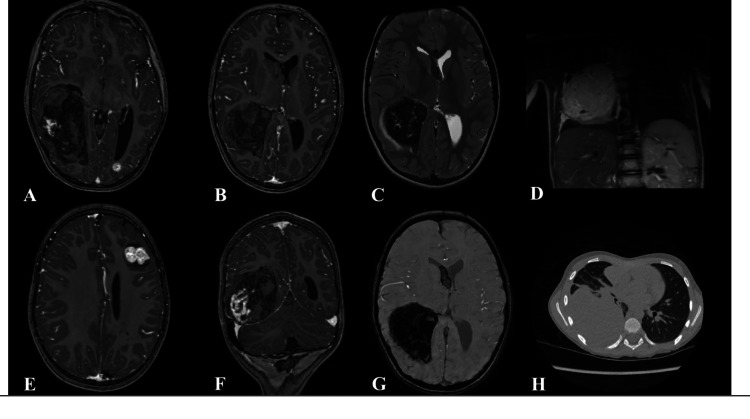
Magnetic resonance imaging (MRI) of the brain and computed tomography (CT) of the thorax demonstrating multiple supratentorial metastatic lesions with extensive intratumoral and intraventricular hemorrhage. A, B, E Axial T1-weighted images with contrast enhancement and F coronal T1-weighted image with contrast enhancement show multiple enhancing metastatic masses with hemorrhagic components. C Axial T2 FSE sequence and G SWI sequence visualize the intraventricular blood products and surrounding oedema. D Coronal T2-weighted image of the thorax image and H axial CT-thorax images showing large multilobed right-sided pulmonary mass
